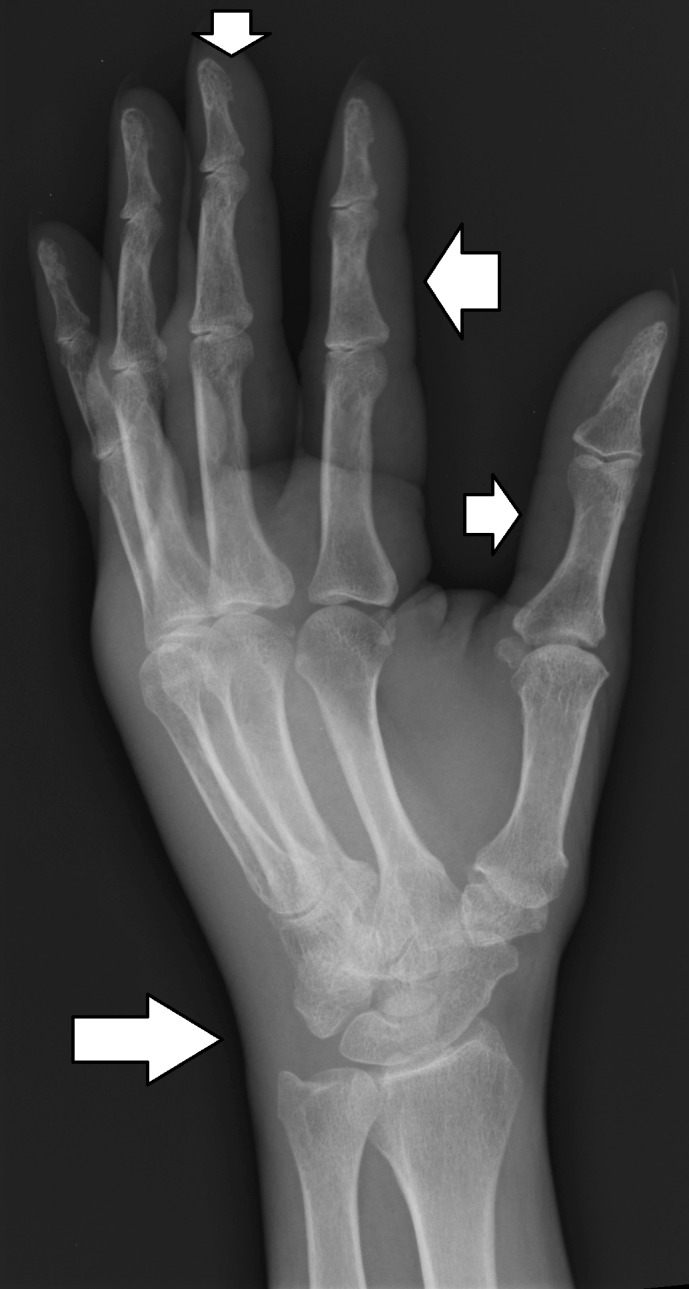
X-ray of the wrist joint showing soft-tissue swelling in the hand and at the wrist joint
